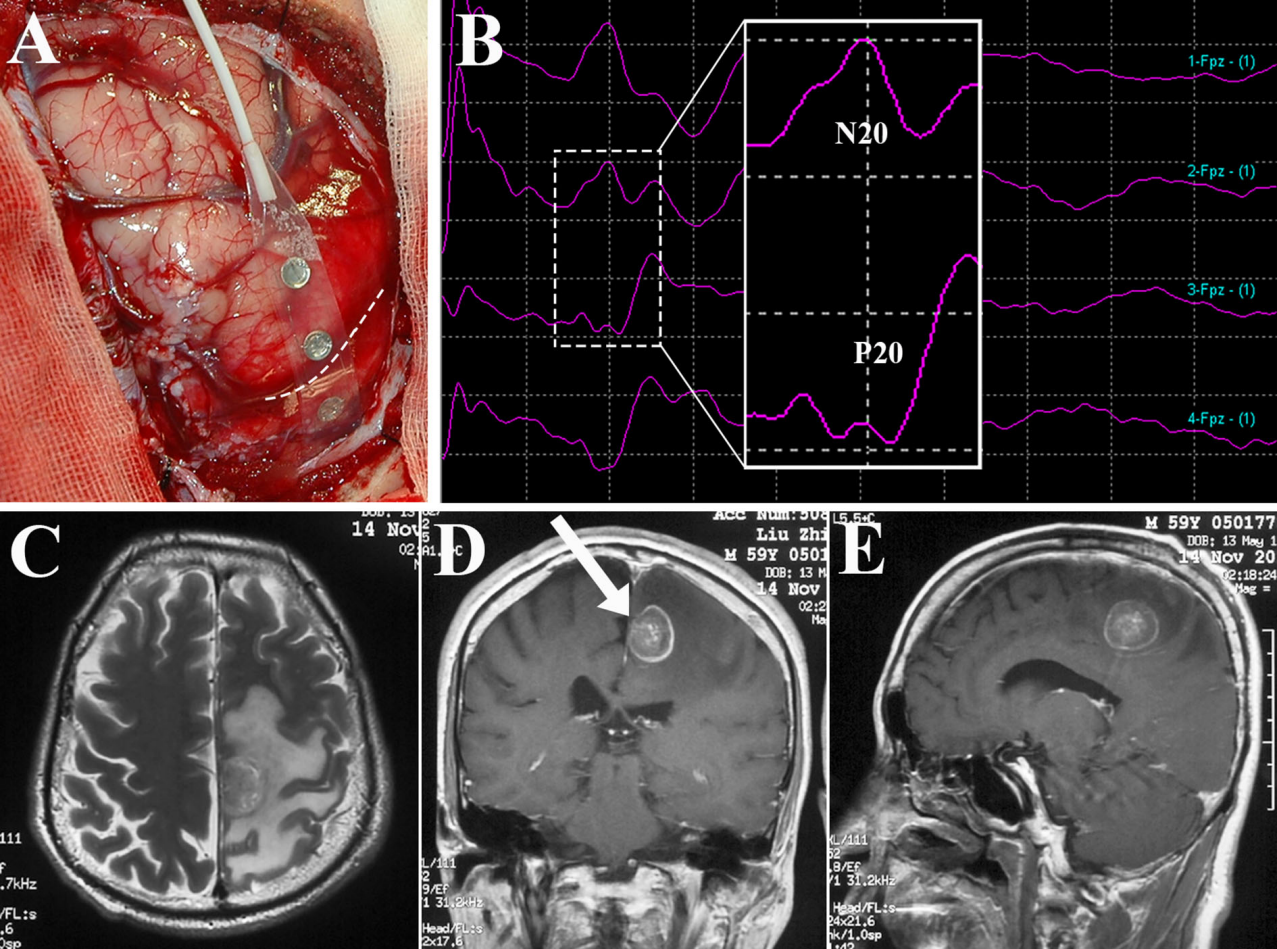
Identification of the central sulcus and sensorimotor cortex using brain mapping. Subdural electrode strip was placed over the perirolandic region (A) to record SEPs, and N20-P20 phase reversal (B) gave information about the site of the central sulcus (dotted line in A). After direct motor cortex mapping, BM allocated within the left paracentral lobule as depicted in axial T2-weighted MRI (C) and contrast sequence in the coronal (D) and sagittal planes (E) was removed *via* the contralateral transfalcine approach (arrow in D). SEPs, somatosensory evoked potentials; MRI, magnetic resonance imaging.
